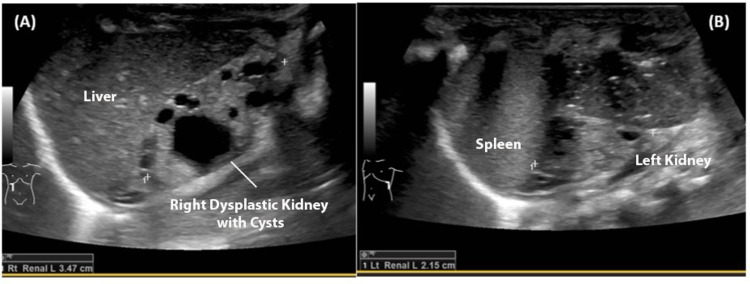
Ultrasound images of the right (A) and left (B) dysplastic kidneys.
